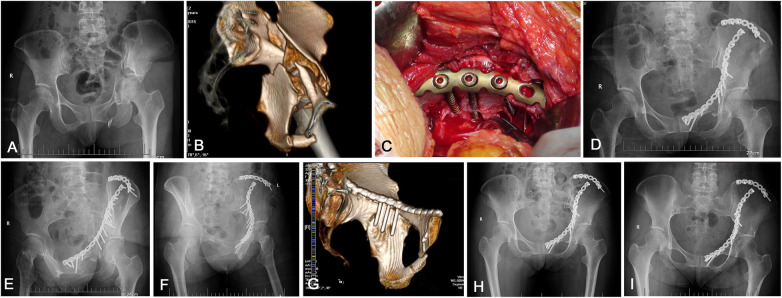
An example showing the special contoured pelvic brim reconstruction titanium plate combined with the quadrilateral screws technique was used for a complicated acetabular fracture involving the QLP. (A) Preoperative AP x-ray of the pelvis shows a displaced both-column acetabular fracture pattern with protrusion following a traffic accident in a 38-year-old female. (B) Preoperative computer tomography (CT) scan with 3D-multiplanar reconstruction. (C) Intraoperative clinical photograph showing reduction and fixation was completed, three quadrilateral screws were placed through the middle segment holes of the contoured plate above the QLP, the screws partially (the 1/3–1/2 transverse diameter) entering the medial bone lamella of QLP. (D–F) Postoperative AP, obturator oblique and iliac oblique views demonstrating the fracture was anatomically reduced and stable. (G) Postoperative CT scan with 3D reconstruction demonstrating three quadrilateral screws were placed on the surface of medial wall, it were distributed as a raft construct, the strong holding force was provided by the special pre-contoured plate to resist the medial displacement of QLP. (H) After 3 months, the fracture had completely united and the reduction was maintained. (I) The AP view after 3 years showing a good radiological result with no evidence of osteoarthritic change.
